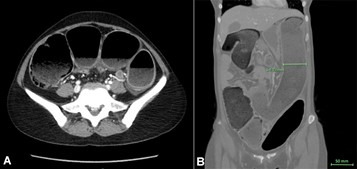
Abdominal computerized tomography (CT) scan with intravenous contrast. Results A and B showed extensive colonic intramural air (pneumatosis intestinalis) prominently involving the ascending colon with significant and potentially life-threatening severe dilation of the entire colon, maximally measuring 6.78 cm in diameter without evidence of free air.
